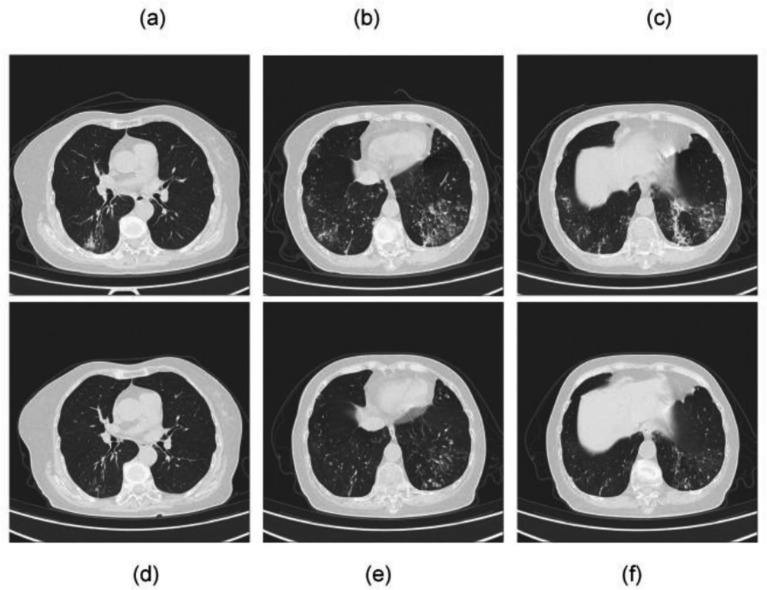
Chest CT scans of Case 1. Prior to treatment initiation: (a–c) multiple ill-defined focal consolidations are observed, accompanied by diffusely increased lung markings and ground-glass opacities in both lungs. One month after treatment: (d–f) the previously noted consolidations have significantly decreased in size and density, with partial resolution of ground-glass opacities.
